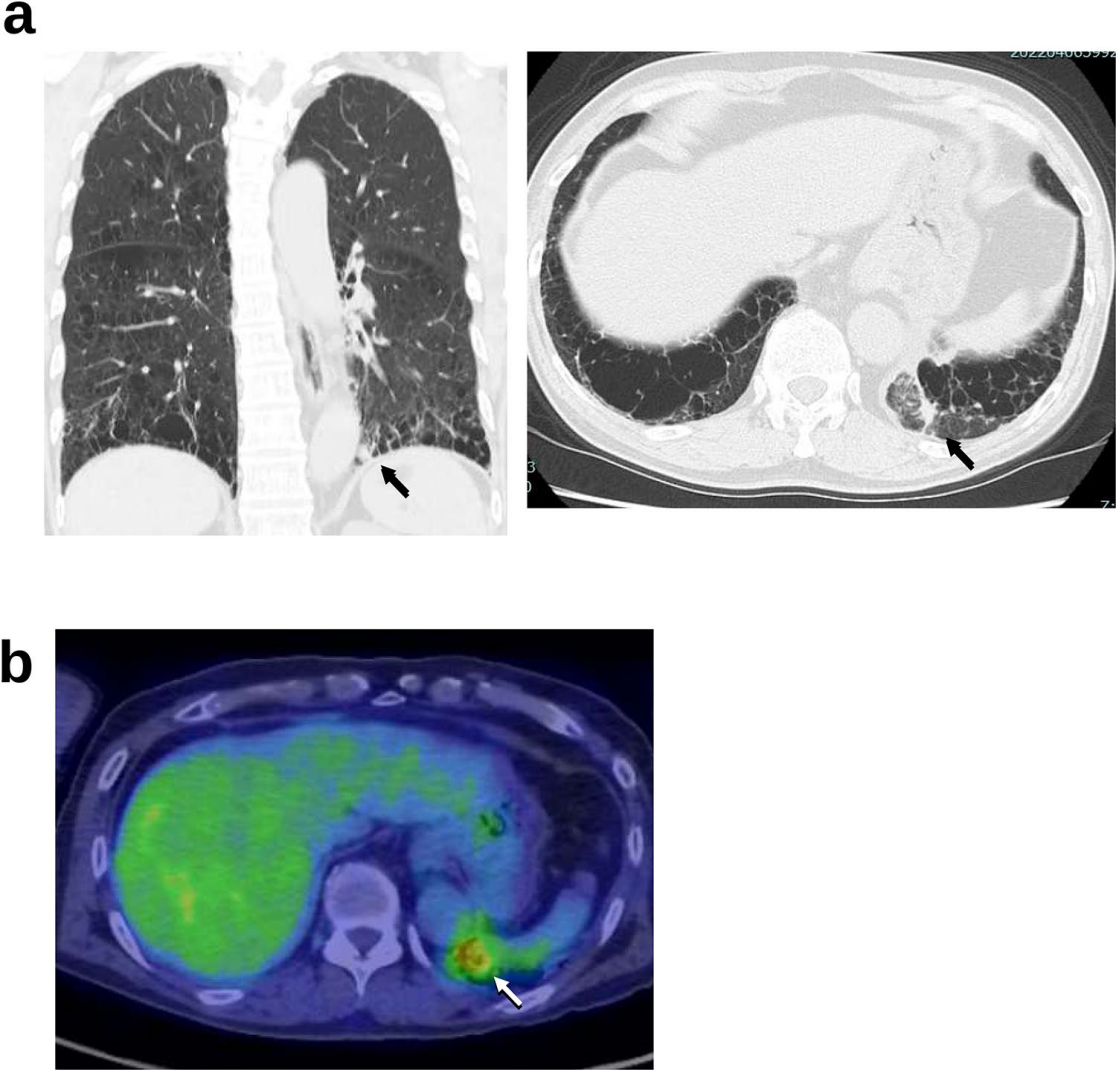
Clinical findings. Computed tomography revealed a nodule with the greatest diameter of 11 mm in the left lower lobe (a, black arrow), and positron emission tomography detected an accumulation of fluorodeoxyglucose in the same area (b, white arrow)
